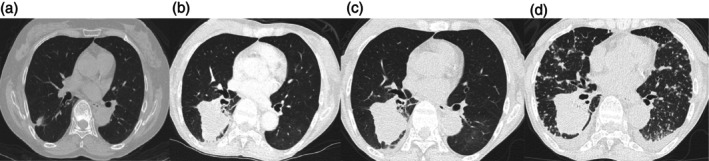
Computed tomography scans at initial diagnosis (a), at the time of recurrence after 11 years (b), after 3 months of osimertinib treatment (c), and at 3 months after osimertinib discontinuation (d).
